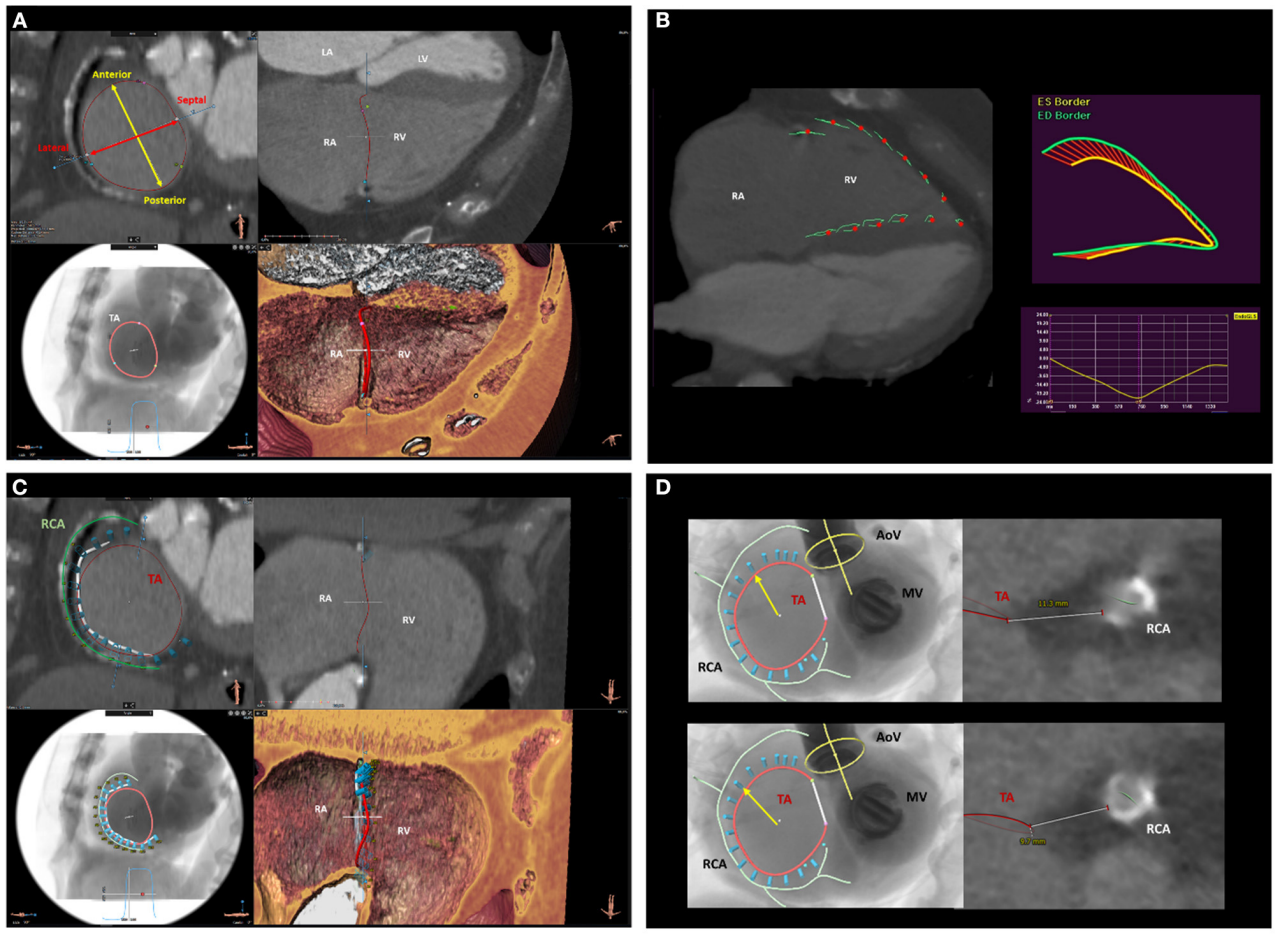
Multi-detector row computed tomography assessment of the tricuspid valve and surrounding structures. (A) displays the MDCT assessment of TA anatomy and dimensions. (B) shows the functional assessment of the RV using computed tomography feature-tracking longitudinal strain. (C) shows the spatial relation of the TA (red circle) with the RCA (green line). This is an important information to assess the feasibility and plan the location where to implant the anchors (light blue cylinders) of the Cardioband device. (D) shows the assessment of the distance between the RCA and the TA on two predicted positions where the anchors of the Cardioband device will be placed. LA, left atrium; LV, left ventricle; MDCT, multi-detector row computed tomography; RA, right atrium; RCA, right coronary artery; RV, right ventricle; TA, tricuspid annulus.
